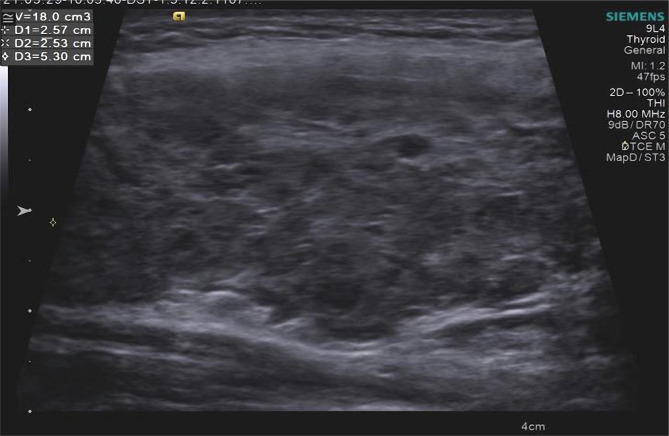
TG sonogram of patient K., 45, with DTG. Heterogeneity of the parenchyma echostructure due to areas of reduced and increased echogenicity.
